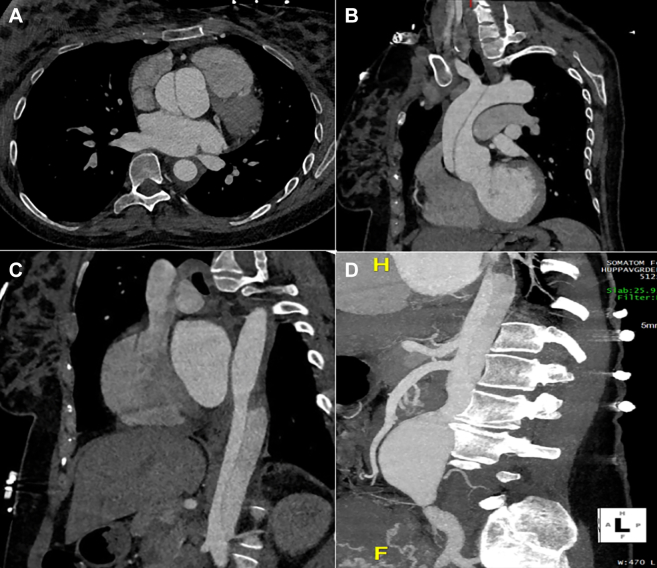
CTA on Postpartum Day 3 Demonstrating New Debakey I Aortic Dissection (A and B) The aortic dissection originated at the level of the aortic root, and (C and D) it extended distally to the infrarenal abdominal aortic aneurysm. CTA = computed tomography angiography.
